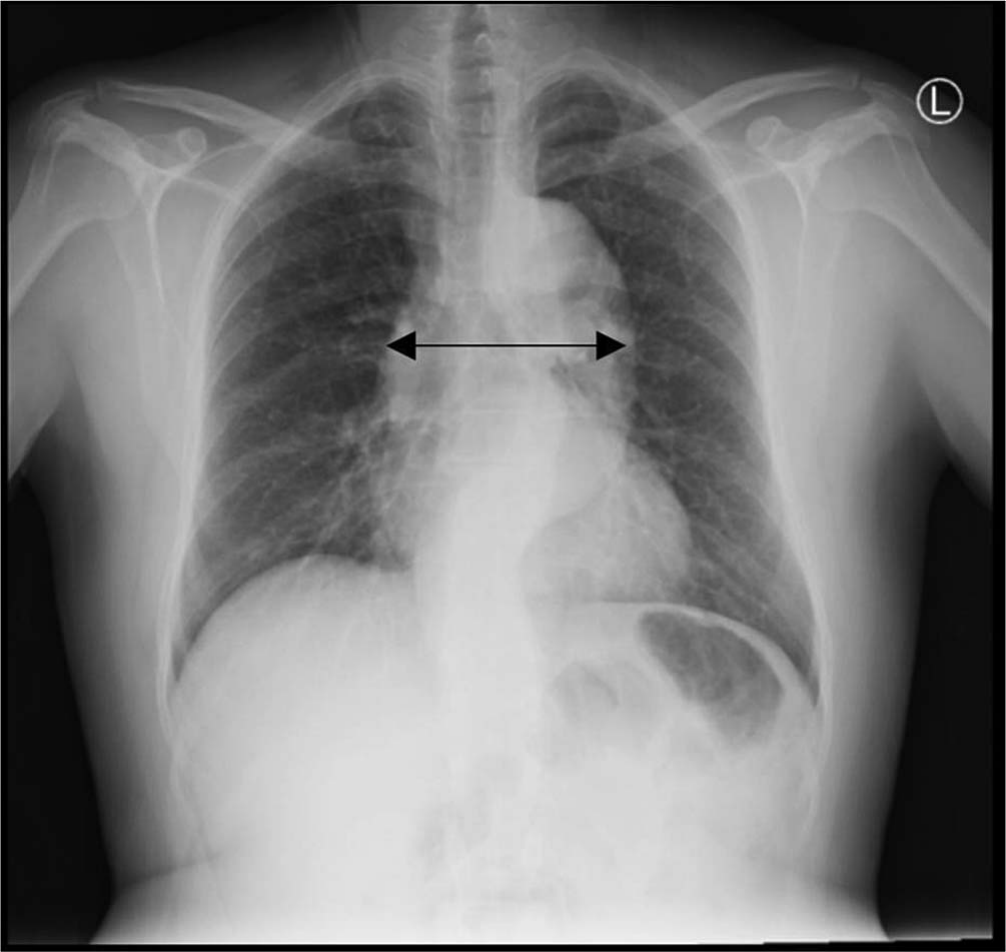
Chest X-ray revealed a widened mediastinum.
